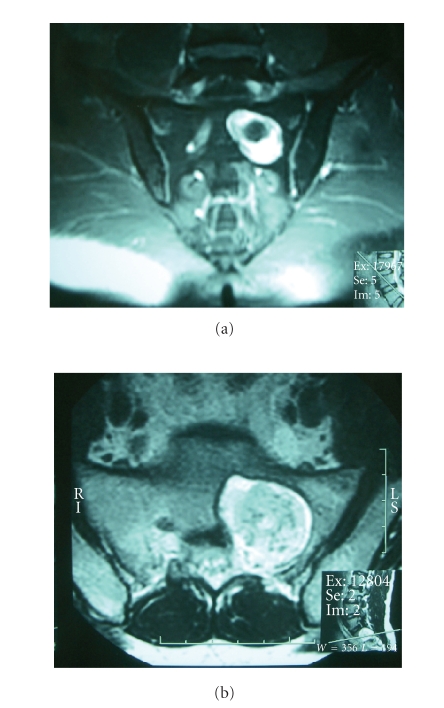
Coronal (Figure 4(a)) and axial (Figure 4(b)) T2-weighted MR images
